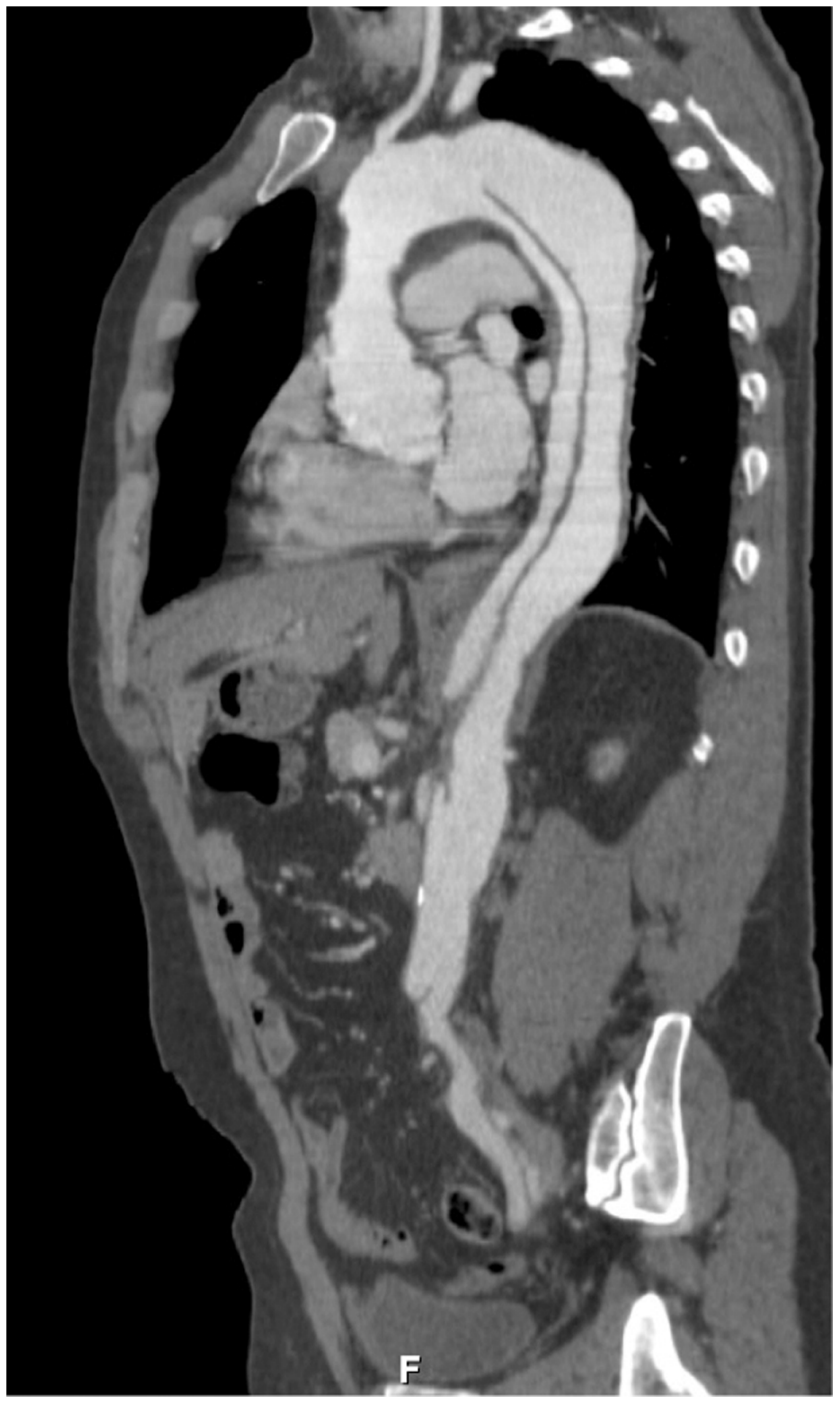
52 year old man presenting with acute chest pain. Contrast enhanced CTA showed a type-b aortic dissection.
